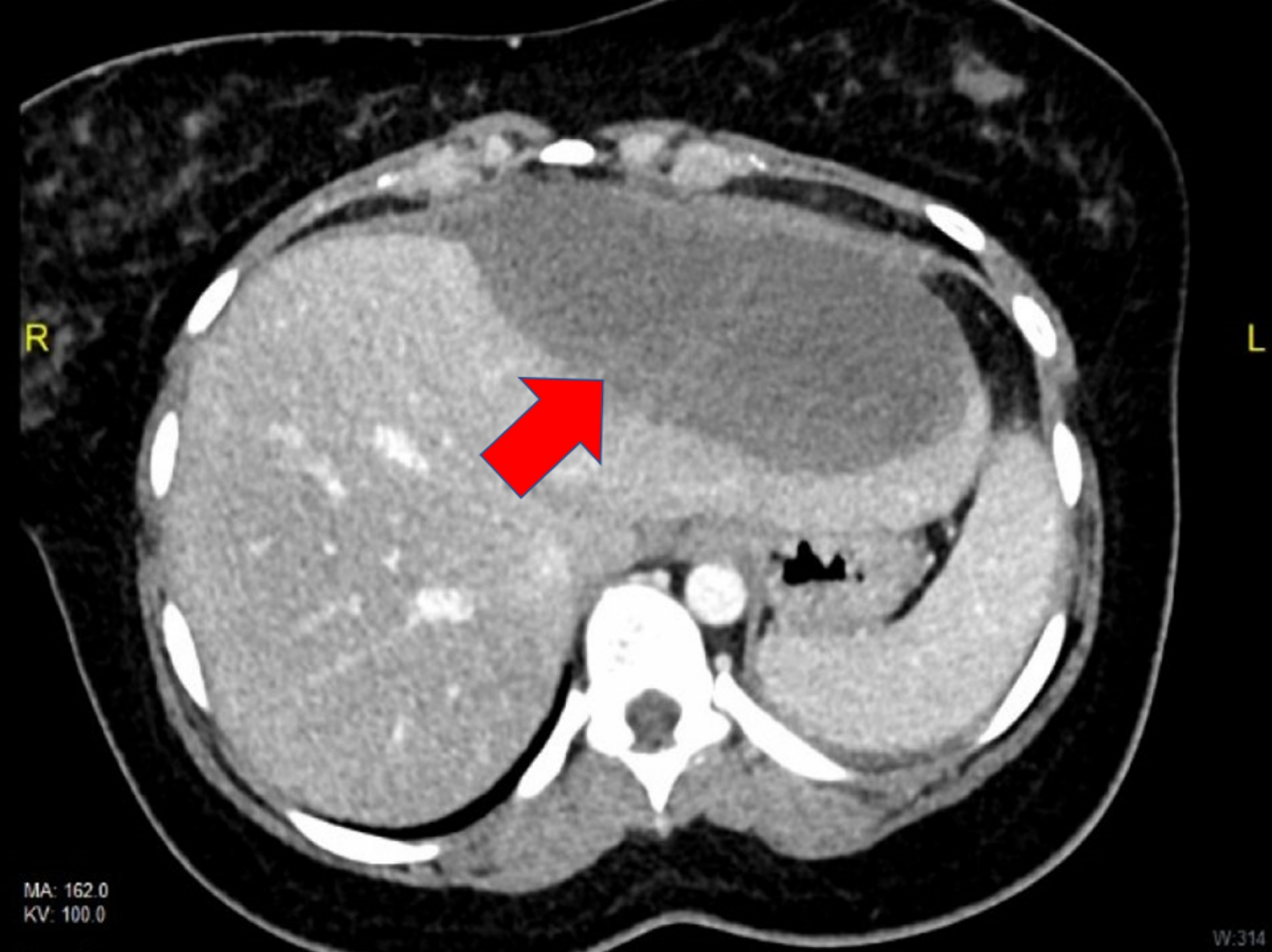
An abdominal CT scan showing a 13.7 x 5.6 x 9.5 cm hepatic subcapsular hematoma
